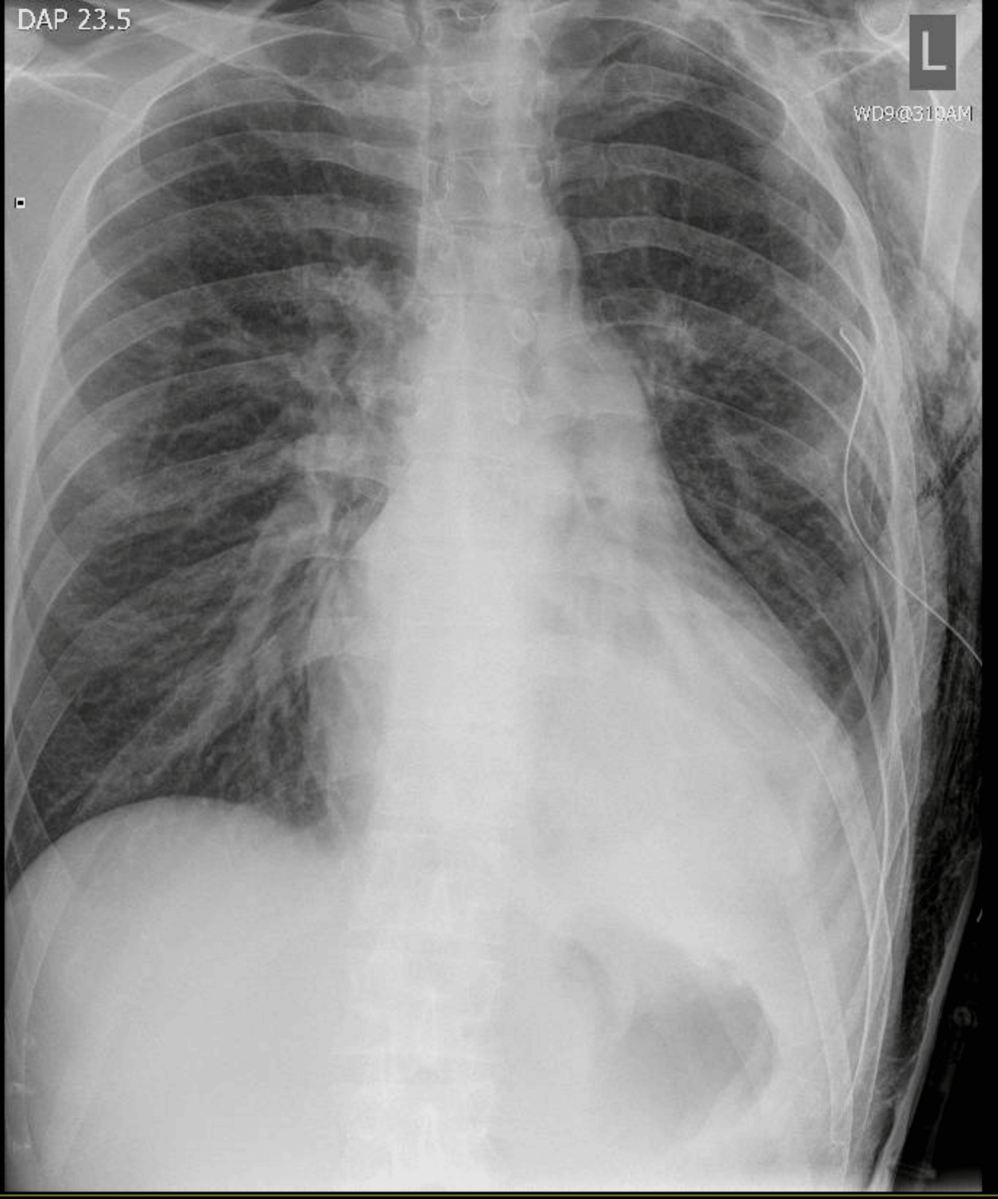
Chest X-ray showed resolution of the left-sided pneumothorax following blood pleurodesis
